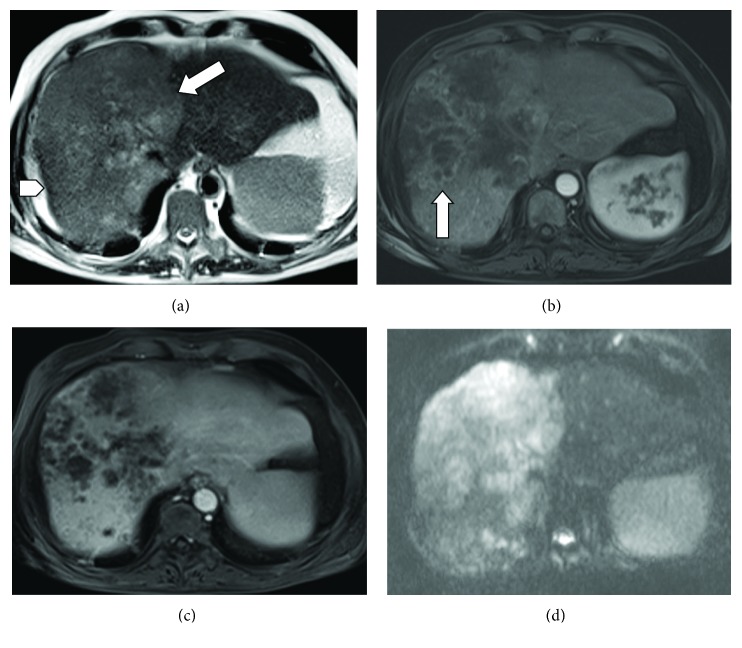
(a) Axial T2-weighted magnetic resonance (MR) image shows a hyperintense mass located in the right hepatic lobe (arrow). There is mild parenchymal atrophy with minimal capsular retraction peripheral to the posterior aspect of the lesion (arrowhead). T1-weighted MR images show irregular, ragged rim enhancement (arrow in (b)) with gradual centripetal enhancement in the portal phase (c). (d) Diffusion weighted image demonstrates high cellularity.
